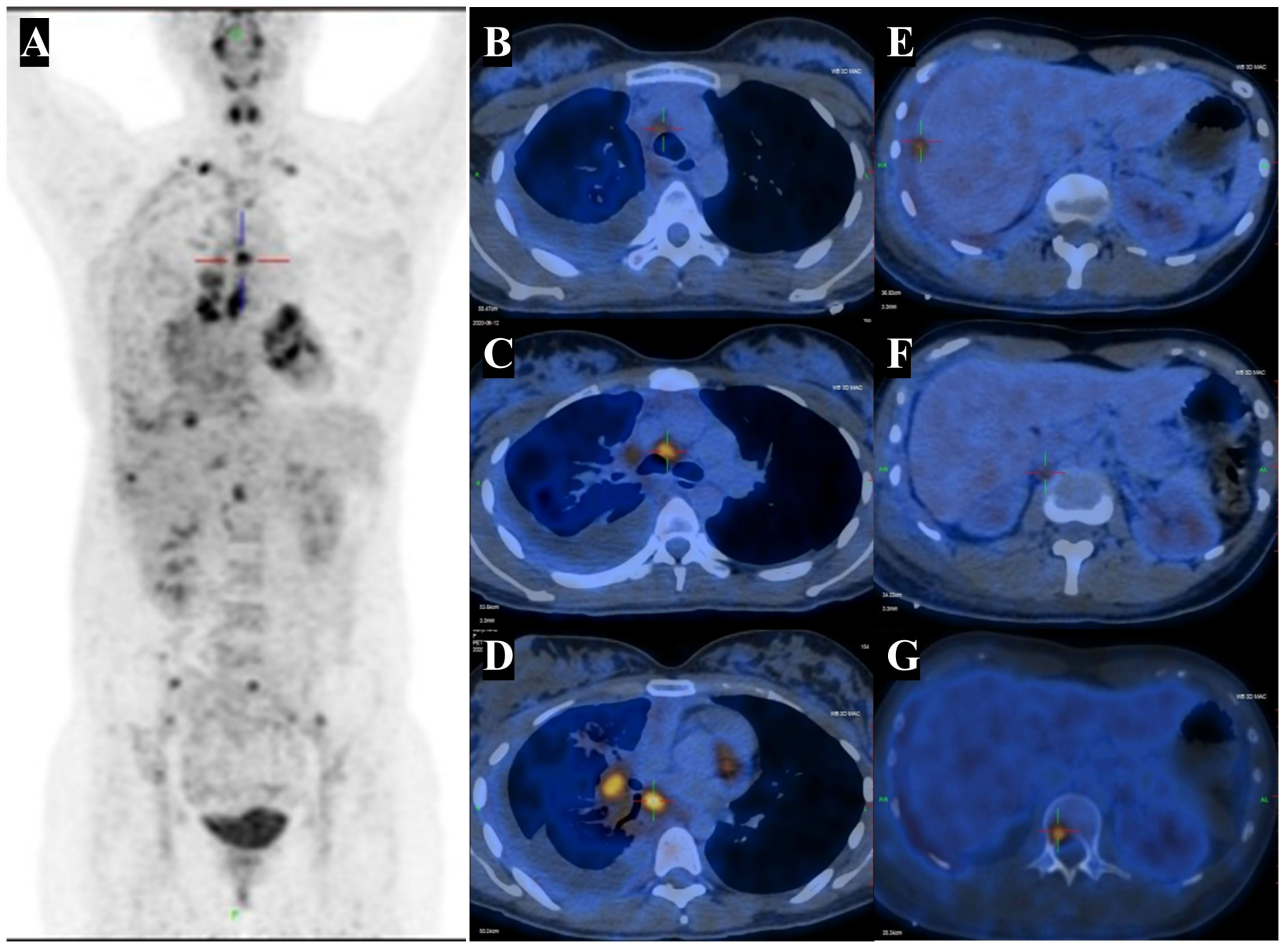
PET-CT highlights multiple hypermetabolic lesions. (A) Fused coronal PET-CT image shows an high metabolic foci in right lung cancer with lymph node, pleural and liver capsule, bone, as well as FDG-avid lesions in the right pleural effusion. (B-D) The right hilar and mediastinal lymph nodes with abnormal FDG uptake. SUVmax 4.4 - 7.8. (E) The abnormal FDG uptake in hepatic capsule with SUVmax 4.6 - 5.6. (F) The abnormal FDG uptake in retroperitoneal lymph nodes with SUVmax 3.4 - 3.6. (G) The abnormal FDG uptake in bone with SUVmax 3.9 - 5.8. CT, computed tomography; PET, positron emission tomography; FDG, fluorodeoxyglucose. SUVmax, maximum standardized uptake value.
